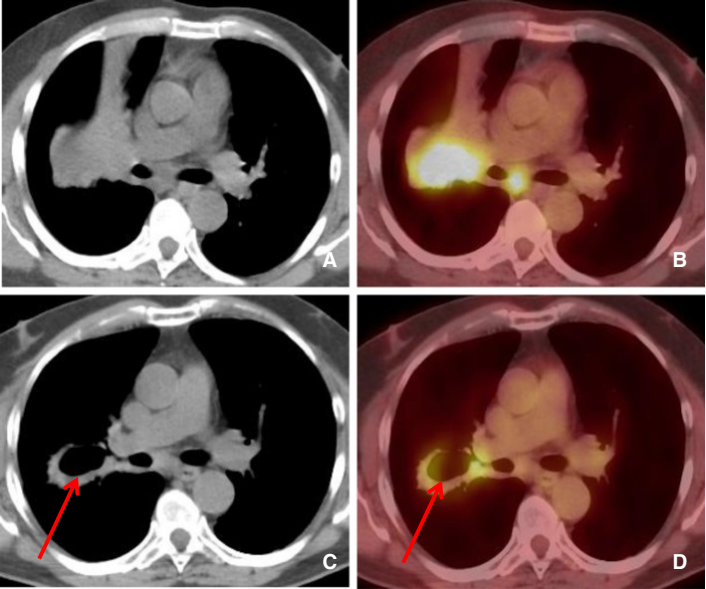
56 year old female with NSCLC treated with Bevacizumab. A-B. Computed tomography and PET-CT demonstrating soft tissue mass in the right middle lobe extending to the right hilar region demonstrating significantly increased tracer uptake. C-D. After 2 cycles of Bevacizumab (Avastin) there has been interval development of pulmonary cavitation within the central portion of the RML mass. PET-CT showing central cavitation and decrease in FDG uptake in the central portion of the mass (arrow). Persistent peripheral soft tissue and right hilar adenopathy are seen.
